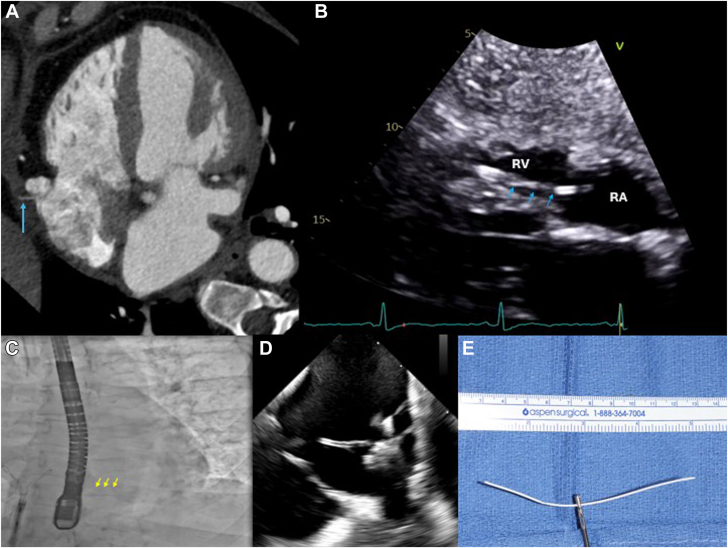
Multimodality imaging and hybrid retrieval of a perforating intracardiac foreign body. (A) Computed tomography demonstrating a 10-cm catheter fragment traversing the right atrium into the right ventricle, with proximal perforation into the pericardial space (arrow). (B) Transthoracic echocardiography showing the linear foreign body crossing the tricuspid valve (arrows). (C) Fluoroscopy during Needle’s Eye Snare attempt (arrows) demonstrating inability to directly visualize the radiolucent foreign body. (D) Intracardiac echocardiography demonstrating Raptor grasping of the fragment. (E) Retrieved intact 10-cm catheter fragment.
